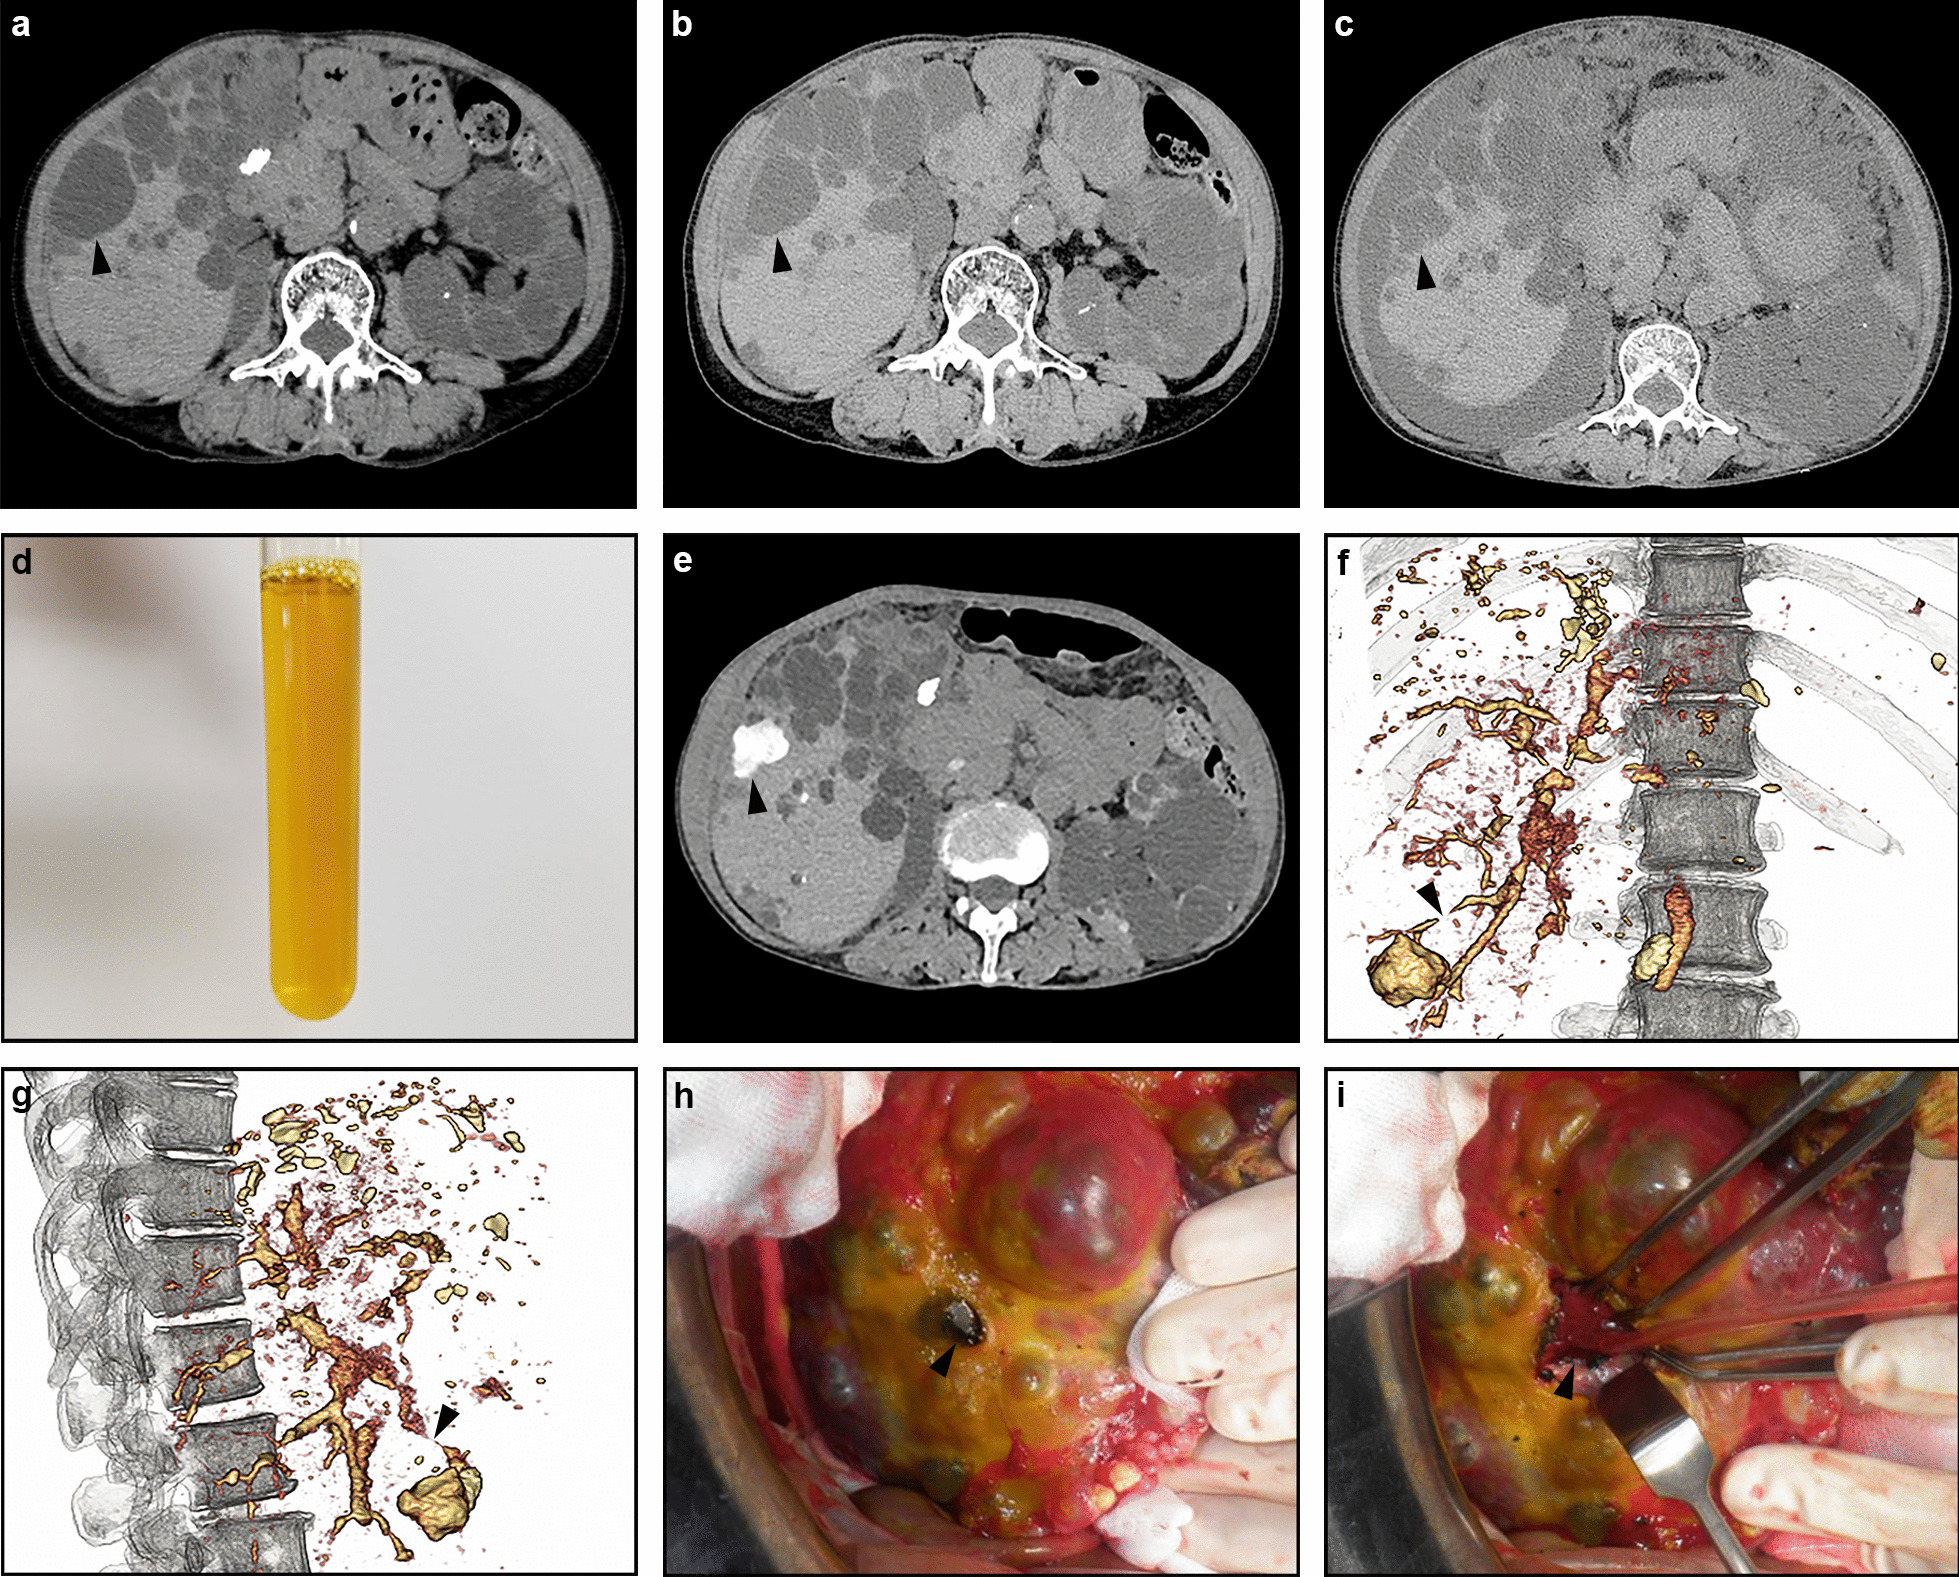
a Abdominal computed tomography (CT) 7 months previously showed gallbladder stones and multiple cysts in the liver and the left kidney. b Abdominal CT at the time of the first admission showed a ruptured liver cyst in the right lobe (arrowhead) while it was not observed seven months previously (arrowhead). c Abdominal CT on the second admission showed that the ruptured cyst (arrowhead) was reduced with massive ascites. d Abdominal paracentesis revealed amber transparent ascites. e Drip infusion cholangiography (DIC)-CT revealed that the ruptured cyst in the right posterior segment S6 of the liver was enhanced with contrast medium (arrowhead). f, g Three-dimensional (3D) views of DIC-CT showed communication between the ruptured cyst and the intrahepatic bile duct (arrowheads, f; front view, g; lateral view). h The surface of the liver became yellowish due to bile leakage and a ruptured necrotic cyst (arrowhead) was observed on the right liver lobe. i After removing the necrotic roof, the orifice (arrowhead) that was the source of the bile leakage was identified
